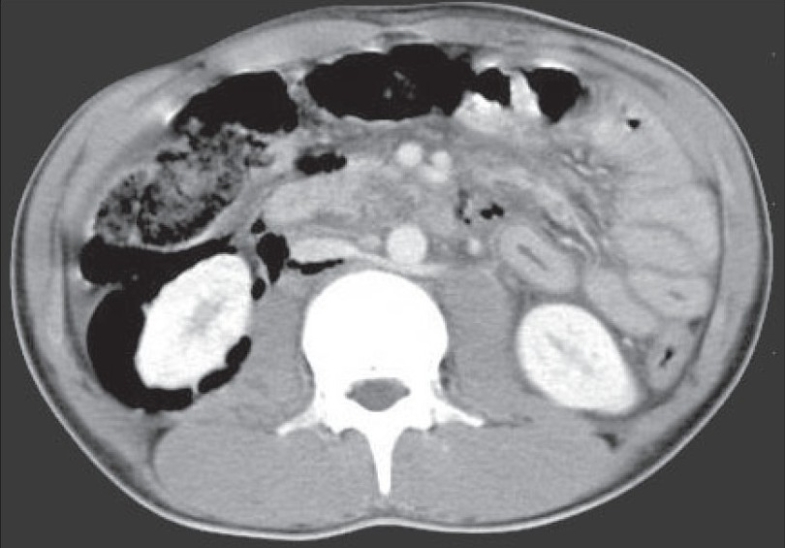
Contrast-enhanced computed tomography axial section at the level of the lower pole of the kidneys showing evidence of foci of air in the mesentery and air collection around the right kidney
